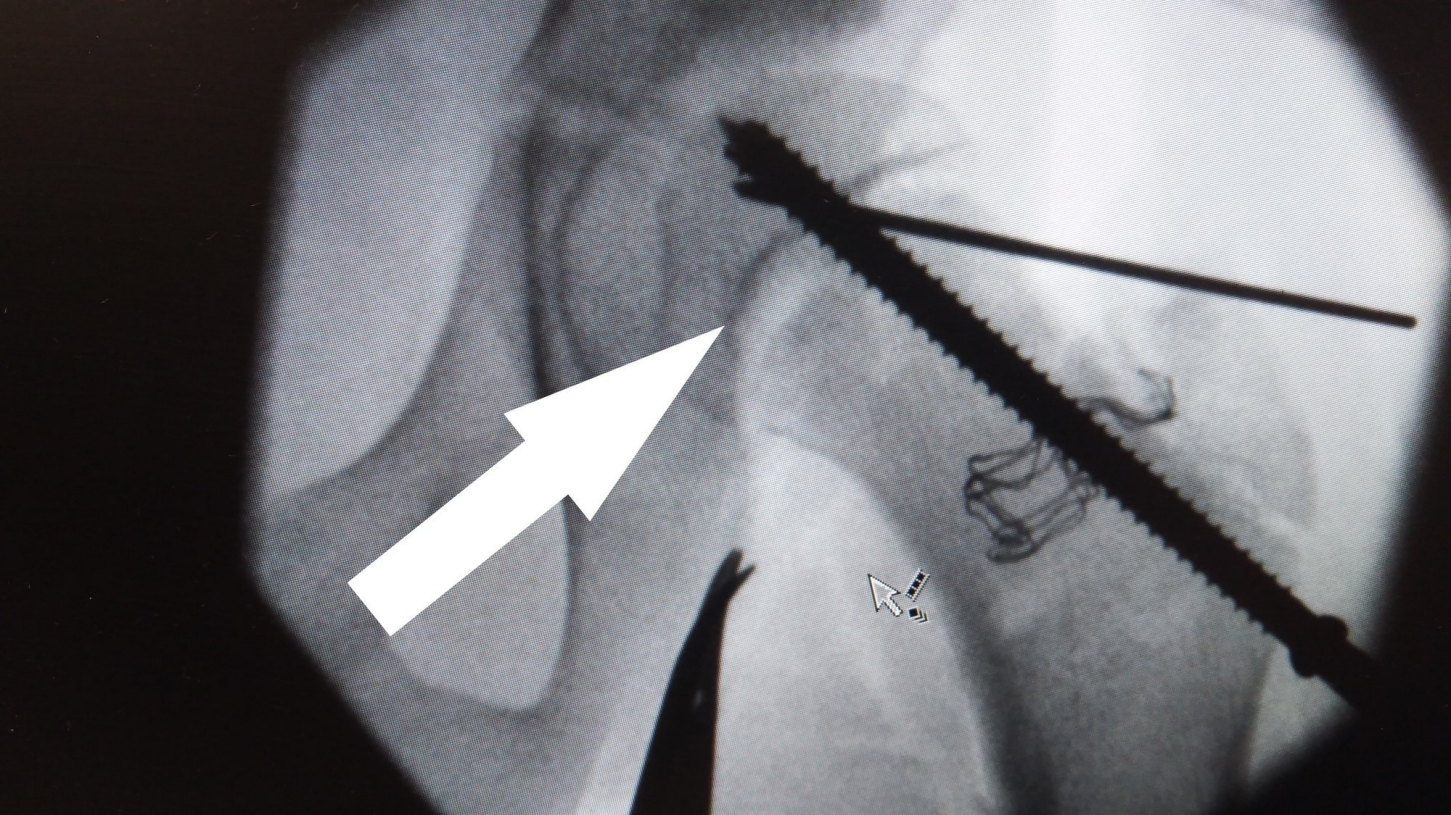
X-ray left hip intra-operative. Final X-ray intra-operative.
